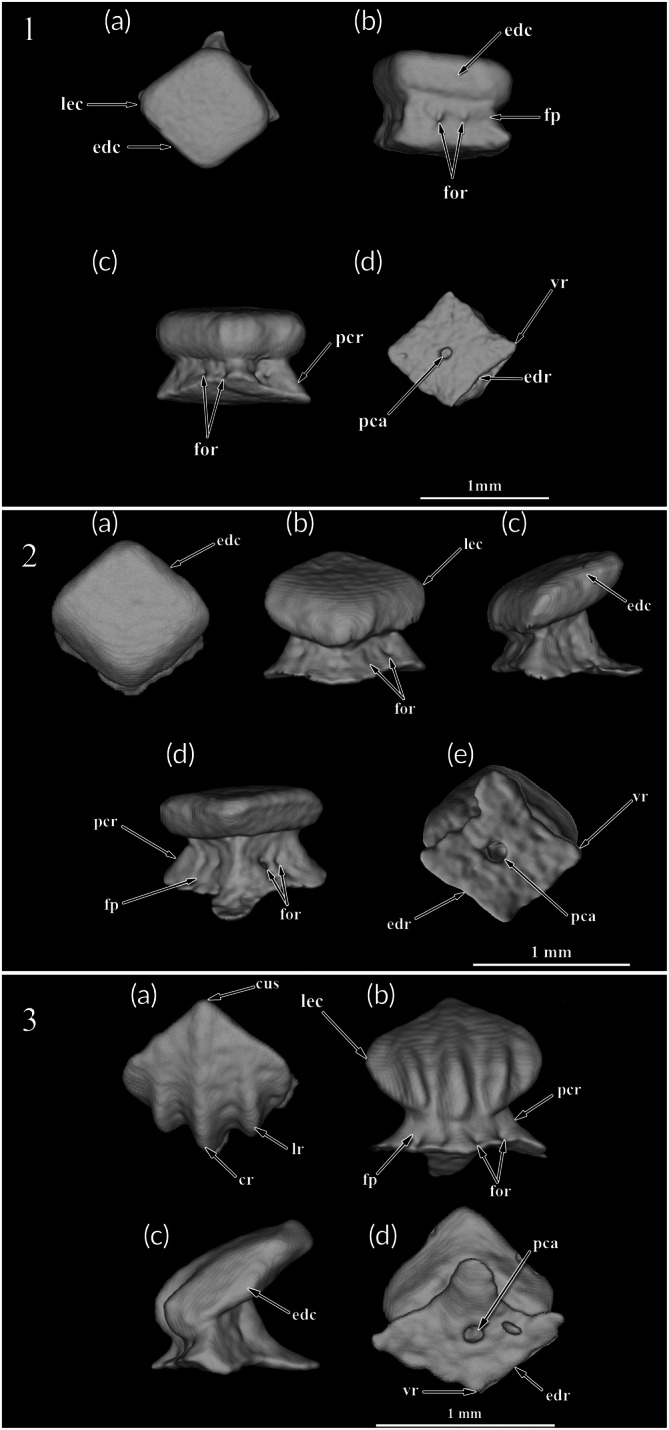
1 = Head type 1 (UFPB 14343 female, 1068 mm PCR). (1a) H1 dorsal view. (1b) H1 frontal view. (1c) H1 posterior view. (1d) H2 ventral view. In (1a, 1d) anterior to bottom; In (1b) anterior to front; In (1c) anterior to back. 2 = head type 2 denticles (UFPB 14343 female, 1068 mm PCR). (2a) H3 dorsal view. (2b) H3 frontal view. (2c) H3 lateral view. (2d) H3 posterior view. (2e) H3 ventral view. In (2a, 2e) anterior to bottom; In (2b) anterior to front; In (2c) anterior to left; In (2d) anterior to back. 3 = Head type 3 (UFPB 14343 female, 1068 mm PCR). (3a) H4 dorsal view. (3b) H4 frontal view. (3c) H4 lateral view. (3d) Ventral view. In (3a, 3d) anterior to bottom; In (3b) anterior to front; In (3c) anterior to left (micro CT‐scan).
